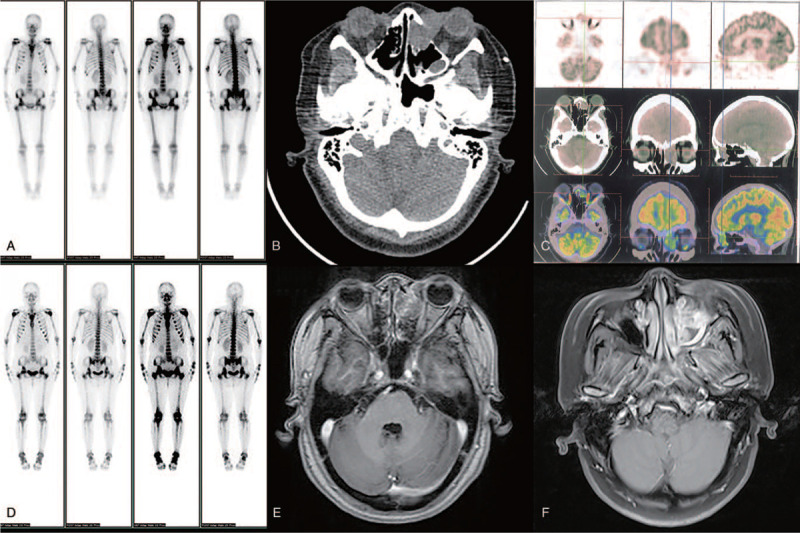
The ECT scan showed that the abnormal distribution of many lesions in the bilateral humerus, sternum, ribs, thoracic vertebrae, lumbar vertebrae, pelvis, and bilateral femurs (A). PET-CT scan showed changes in the left nasal cavity of the patient (B). PET-CT showed increased shadow metabolism of soft tissue density. Its intake of tracer 18F-fluorine deoxyglucose was 6.8 units (C). The ECT crown position of the whole body bone after chemotherapy (D). After chemotherapy, the skull MRI examination axis T1WI showed a slightly lower signal for the tumor. The signal is lower than the brain (E). The MRI of the skull after chemotherapy showed that the axis T2WI showed a tumor signal higher than the brain parenchyma (F).
